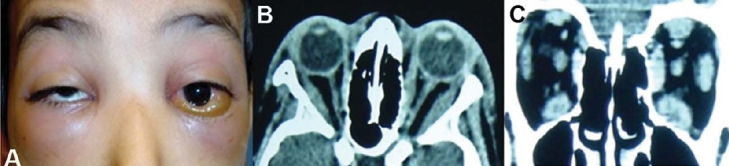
A 10 year old boy presented with fever, headache, !ì vision, chemosis, proptosis, restricted ocular motility anddecreased vision of both eyes (A). A CT-scan (axial and cronal) reveled bilateral extraocular muscle thickening involvingtendons (B and C).
